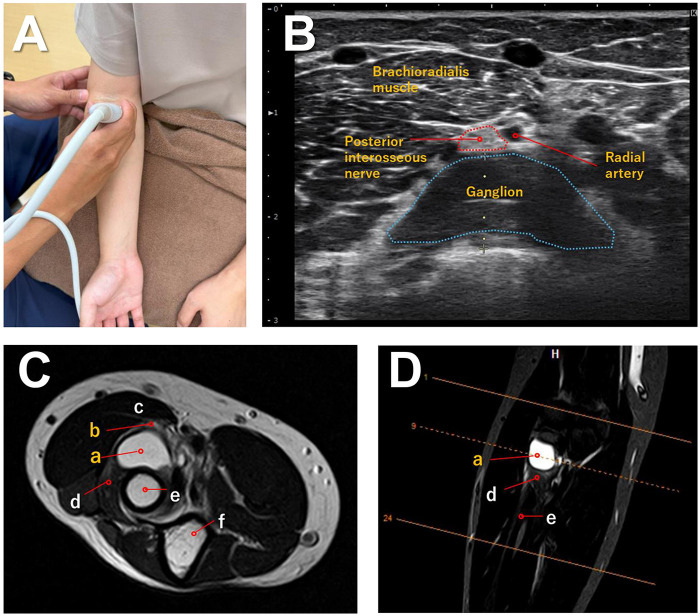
Ultrasound and magnetic resonance imaging of posterior interosseous nerve compression at the proximal forearm. (A) Photograph showing the probe placed over the proximal dorsal forearm with the elbow flexed and the forearm supinated. (B) Short-axis ultrasound image demonstrating the posterior interosseous nerve compressed between the brachioradialis muscle and a large ganglion in the radial tunnel, adjacent to the accompanying vessels. The cross-sectional areas of the nerve and ganglion are outlined, and the posterior interosseous nerve shows a flattened transverse shape compared with the contralateral side. (C) Axial T2-weighted magnetic resonance image at the same level showing the posterior interosseous nerve (a) compressed by a hyperintense ganglion (b) between the brachioradialis muscle (c) and the supinator muscle (d) The radius (e) and ulna (f) are also indicated. (D) Coronal T2-weighted magnetic resonance image demonstrating the ganglion (a) along the course of the posterior interosseous nerve, with the supinator muscle (d) and radius (e) labeled; the dashed line indicates the level of the axial slice shown in (C).
